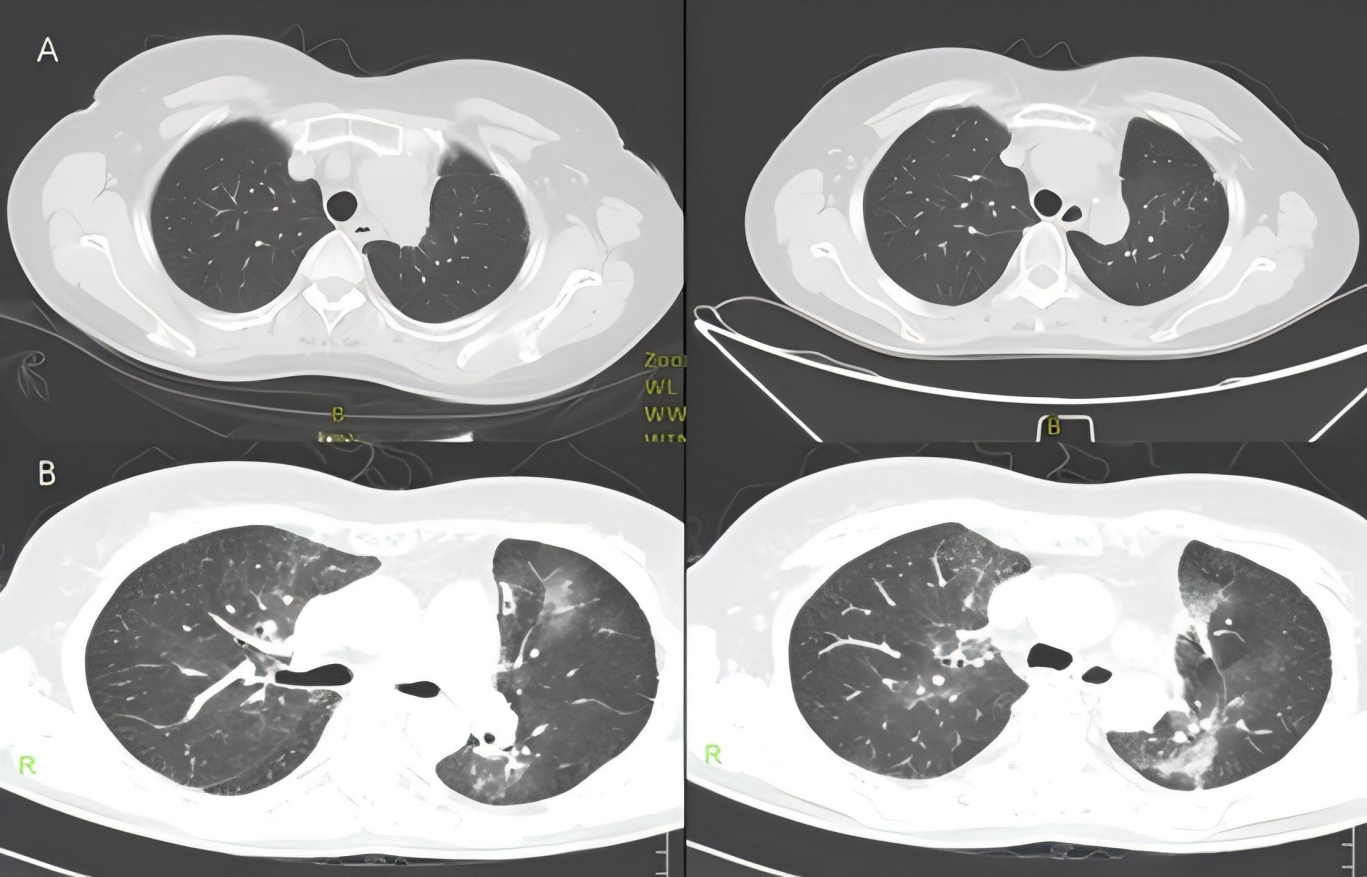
(A) Chest CT showed no obvious symptoms of pneumonia on May 8, 2021. (B) Chest CT showed radiation pneumonitis on May 29, 2021.
